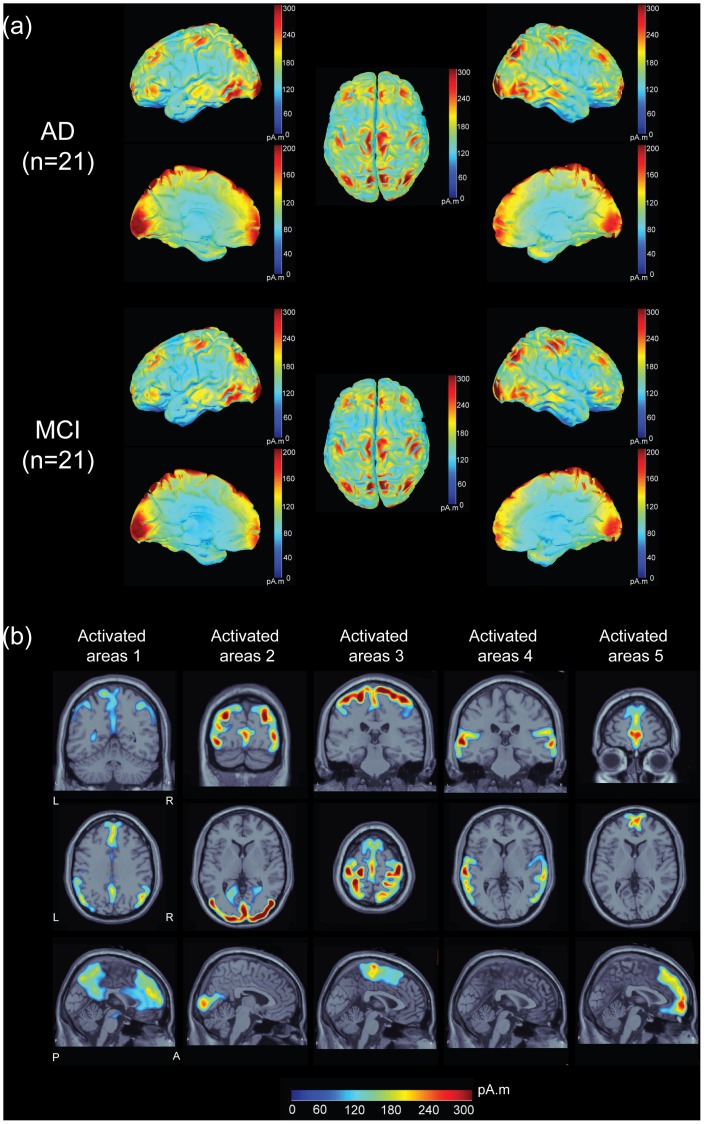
Resting state networks from EEG data. (a) Distributions of averaged cortical activation during an eyes-closed resting-state condition in 21 AD and 21 MCI patients, respectively. (b) 5 activated maps on the sagittal, coronal and axial MR images are shown. The sources values were smoothed after being re-interpolated (the size of the smoothing kernel = 5). The current strength of cortical sources is color coded; large values are represented in red, and small values are in blue.
